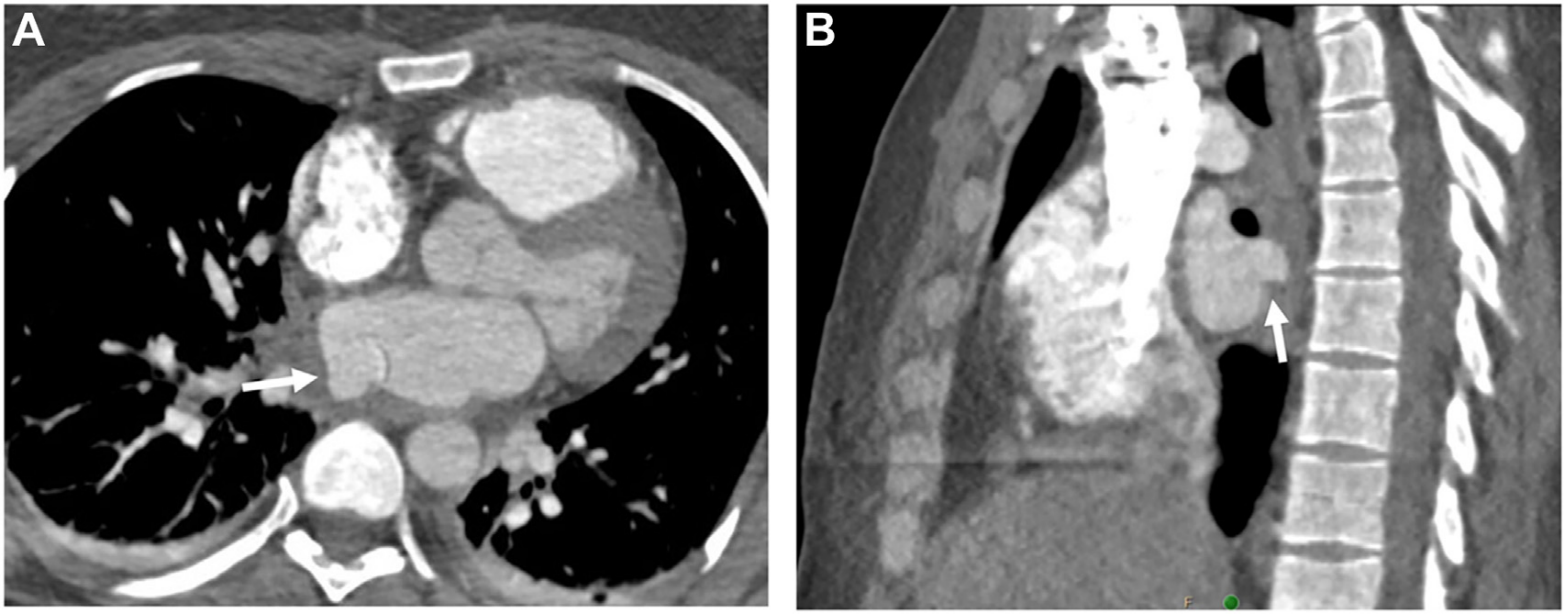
Axial (A) and sagittal (B) views of cardiac computed tomography angiograph demonstrating the left atrial pseudoaneurysm (arrow) and adjacent intrapericardial air.
